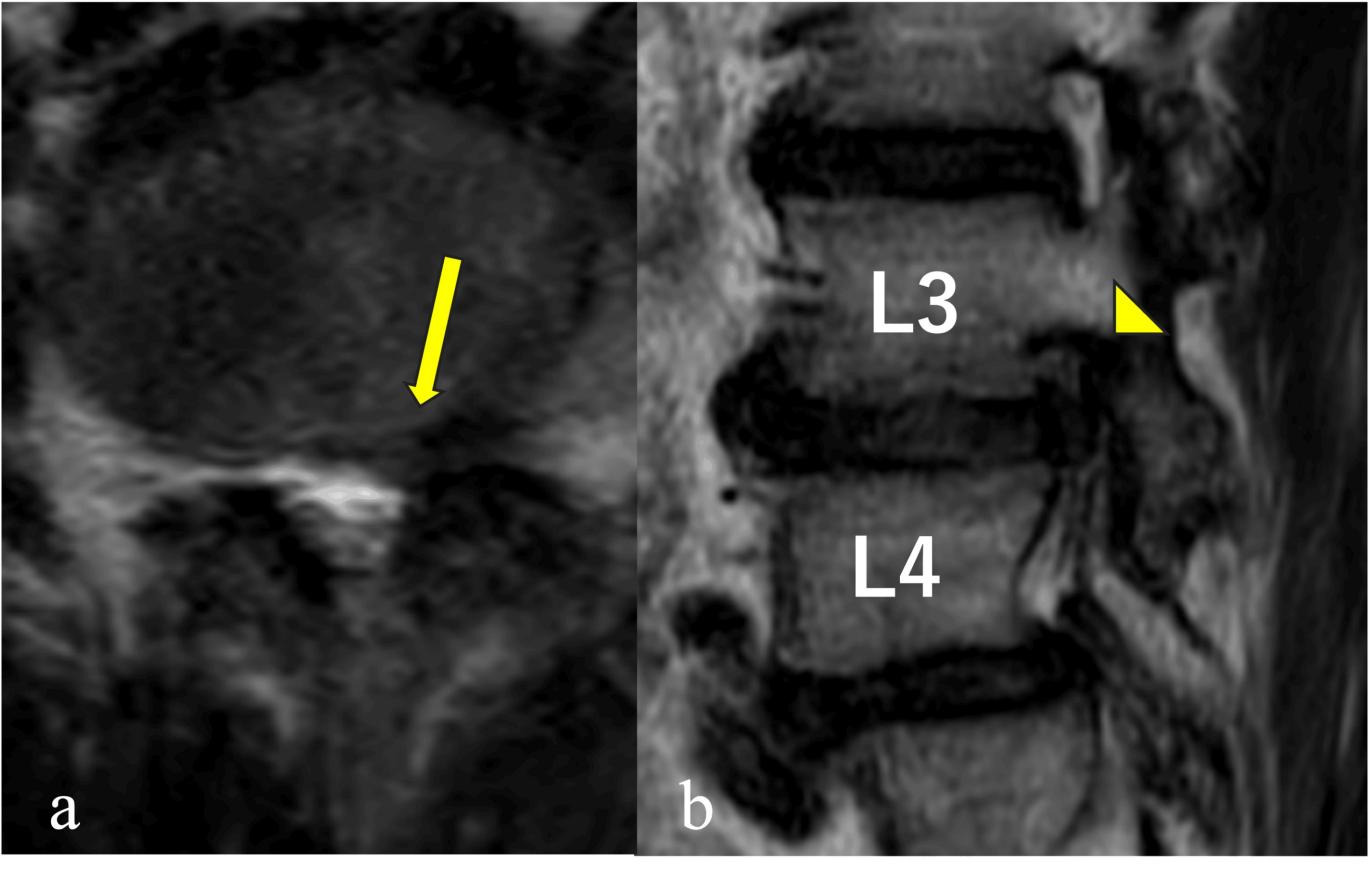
Axial and sagittal MRI findings in case 2 Axial T2-weighted MR image (a) in case 2 shows a foraminal-type lumbar disc herniation at L3/4 (arrow). On the sagittal image (b), the L3 nerve root is displaced cranially (arrowhead).
